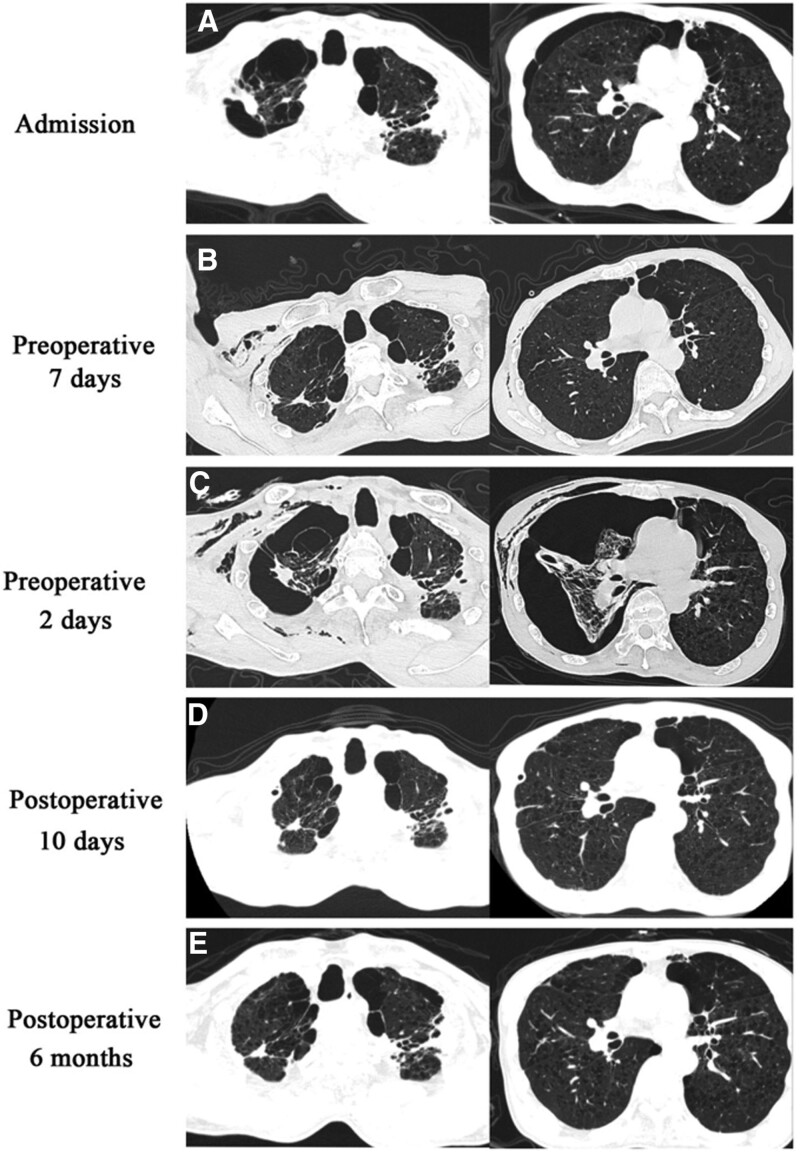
CT details of case 1. (A) On admission, (B) 7 days before operation, also the fifth day of drainage tube placement, (C) 2 days before operation, 2 days after the second drainage, (D) The 10th day after operation, and (E) 6 months after operation.
